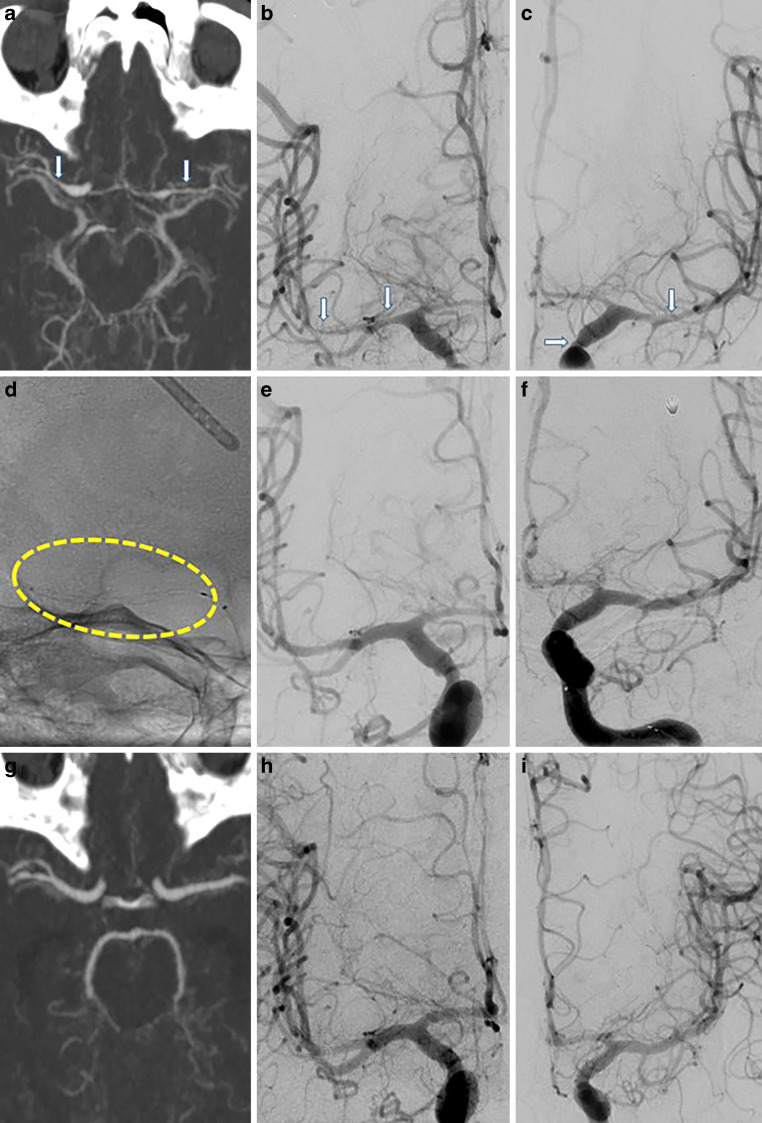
a, b, c Initial CTA (computed tomography angiography) and DSA (digital subtraction angiography) on post-ictus day 9. Vasospasm of M1 and M2 bilaterally (*arrows*). d pRELAX 4–20 mm deployed in M1–M2 segments. e, f DSA 15 min after Stent-ReLACSS (Stent Retraction to relieve Arterial Cerebral vasoSpasm caused by SAH) showing sufficient vasodilatation. g CTA 1 day after EVT (endovascular treatment) with Stent-ReLACSS showing sufficient vasodilatation. h, i Angiogram 15 days after Stent-ReLACSS showing regular vessel lumen and no vessel injury. No other endovascular treatment for CVS had been performed in the meantime for the internal cerebral artery or middle cerebral artery
